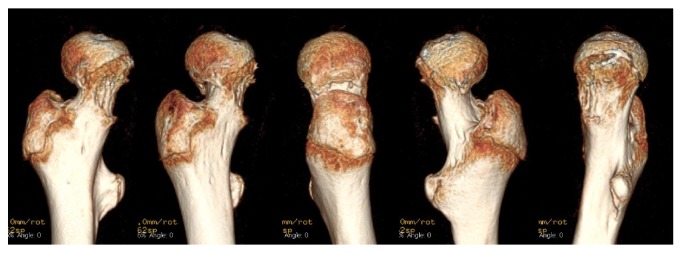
3D CT images showed the right epiphyseal displacement was laterally.
